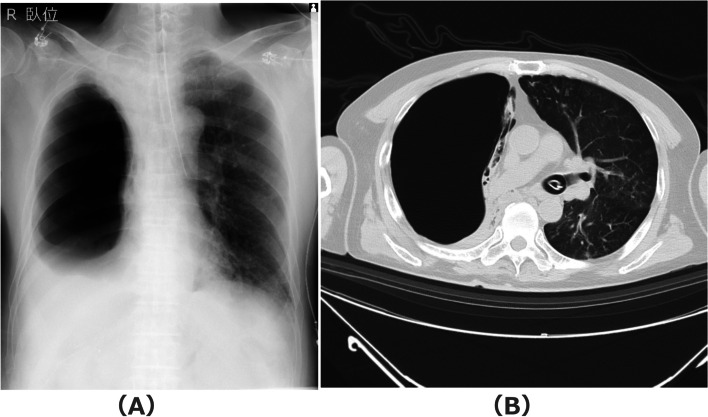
Postoperative chest radiography (A) and computed tomography (B) before extubation. Hyperinflated giant bulla compressed the whole right lung, including the upper lobe, to atelectasis
